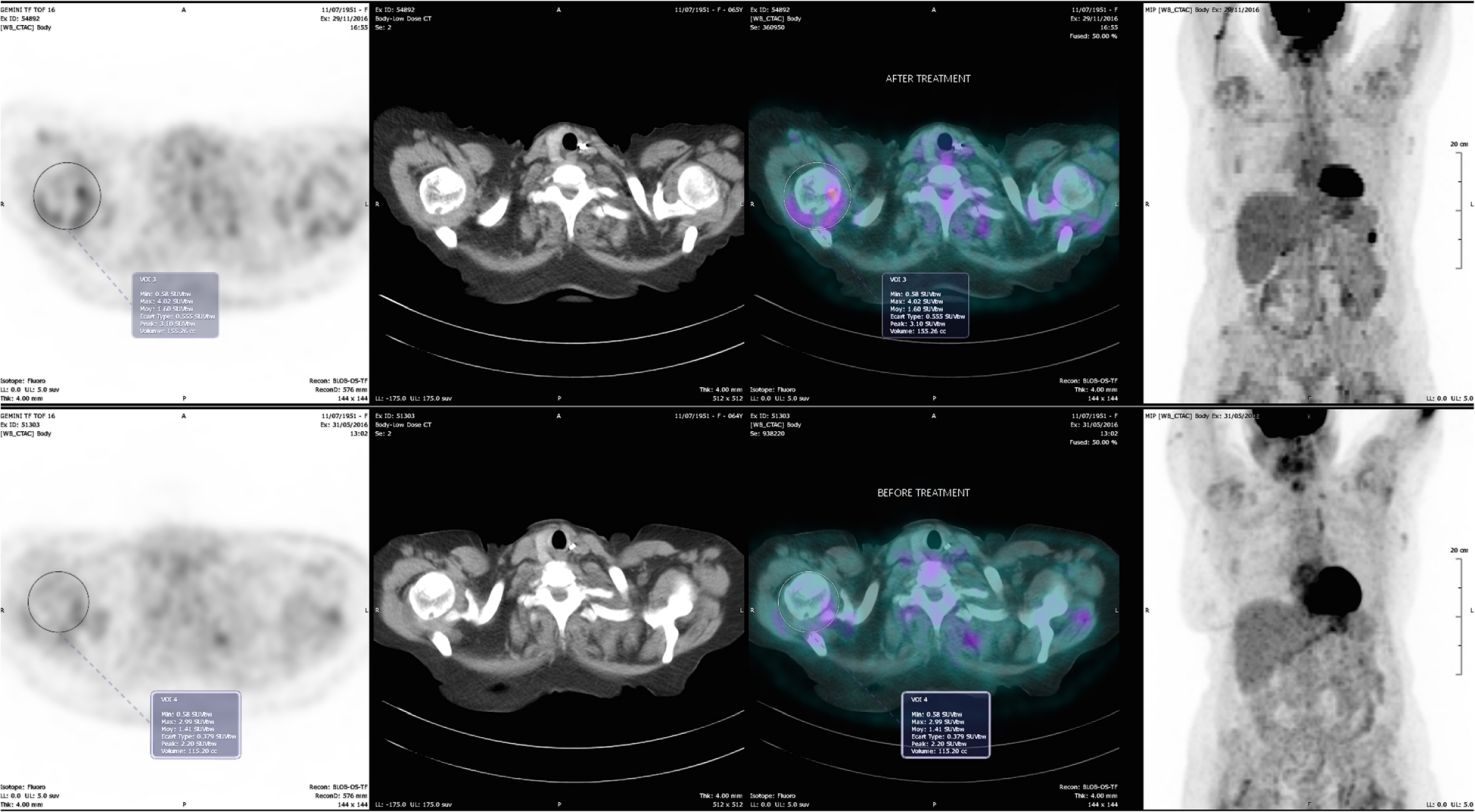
PET scan: upper image: after treatment; lower: before treatment. Hips. Diffuse, metabolically active deposits, specifically in the settings of intense pain. The pattern is consistent with diffuse periarticular hyperfixation. The metabolic activity is moderate, the standardised uptake value (SUV) increases from 3.40 to 4.19 at 6 months
